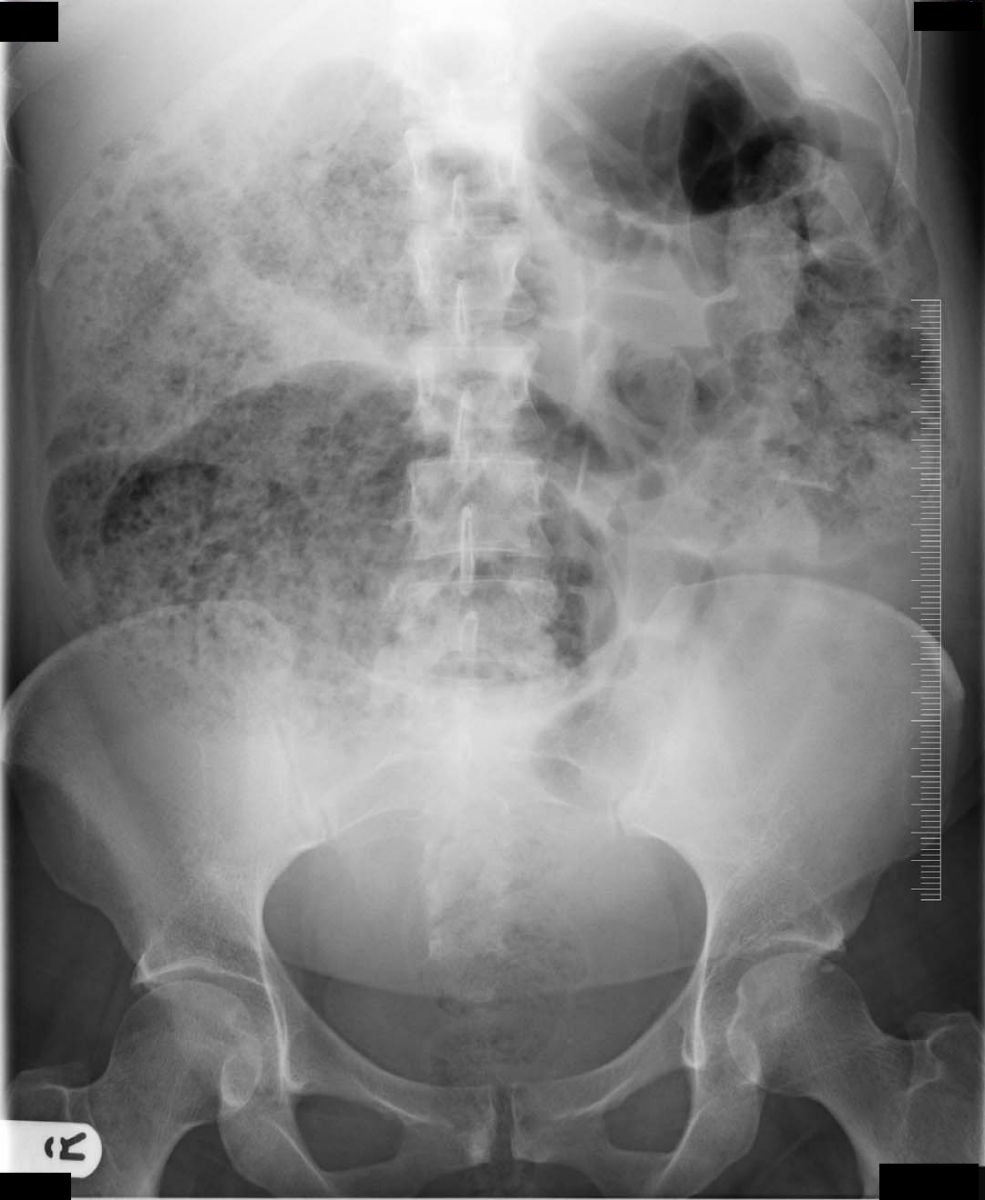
Abdominal radiograph.
